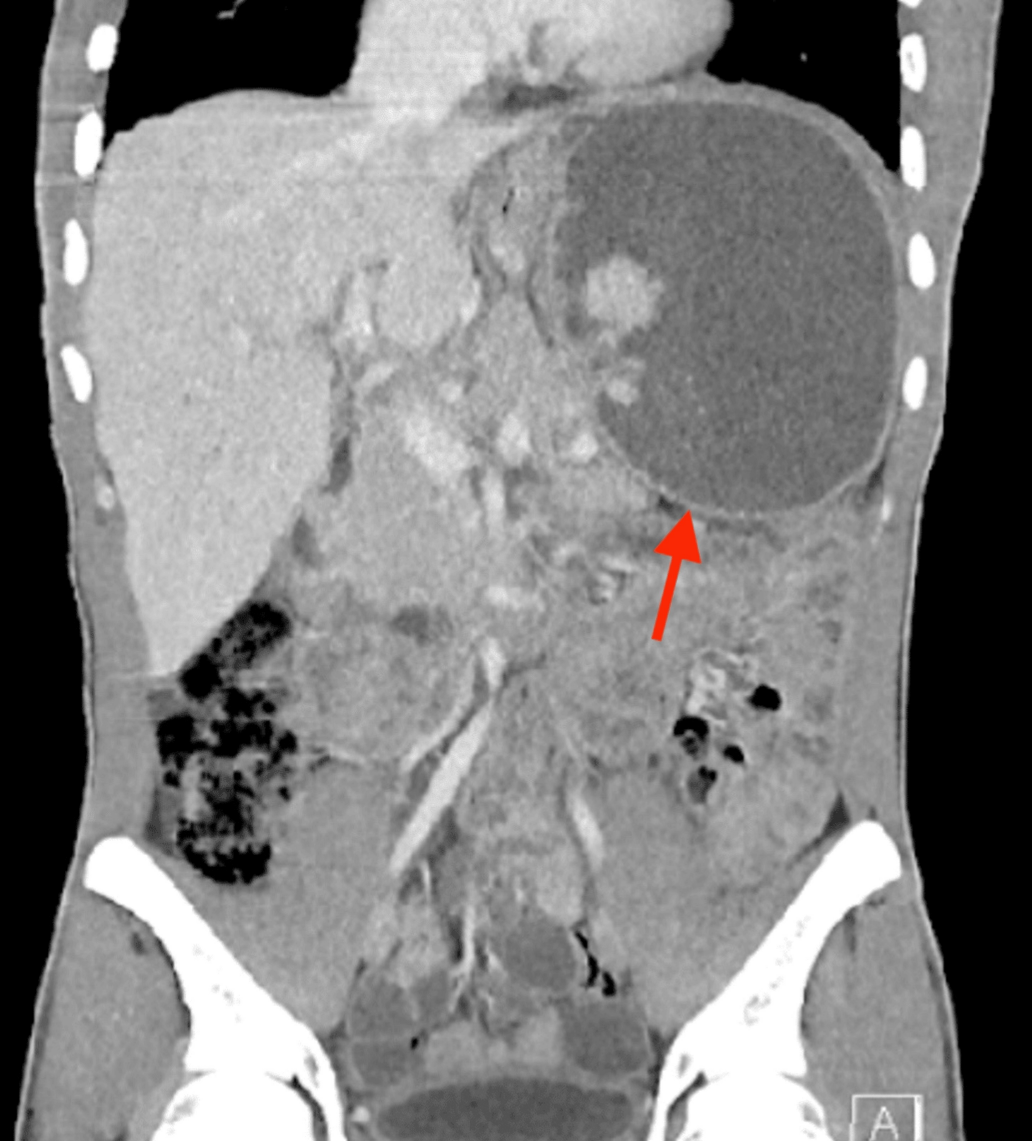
Coronal view CT abdomen/pelvis Coronal view CT abdomen/pelvis showing 12.7 x 7.8 x 14.5 cm heterogeneous lesion with areas of fluid attenuation more laterally in the left upper abdominal quadrant. Multiple hyperattenuating nodular lesions within the greater lesion, which may represent severely atrophic splenic tissue.
